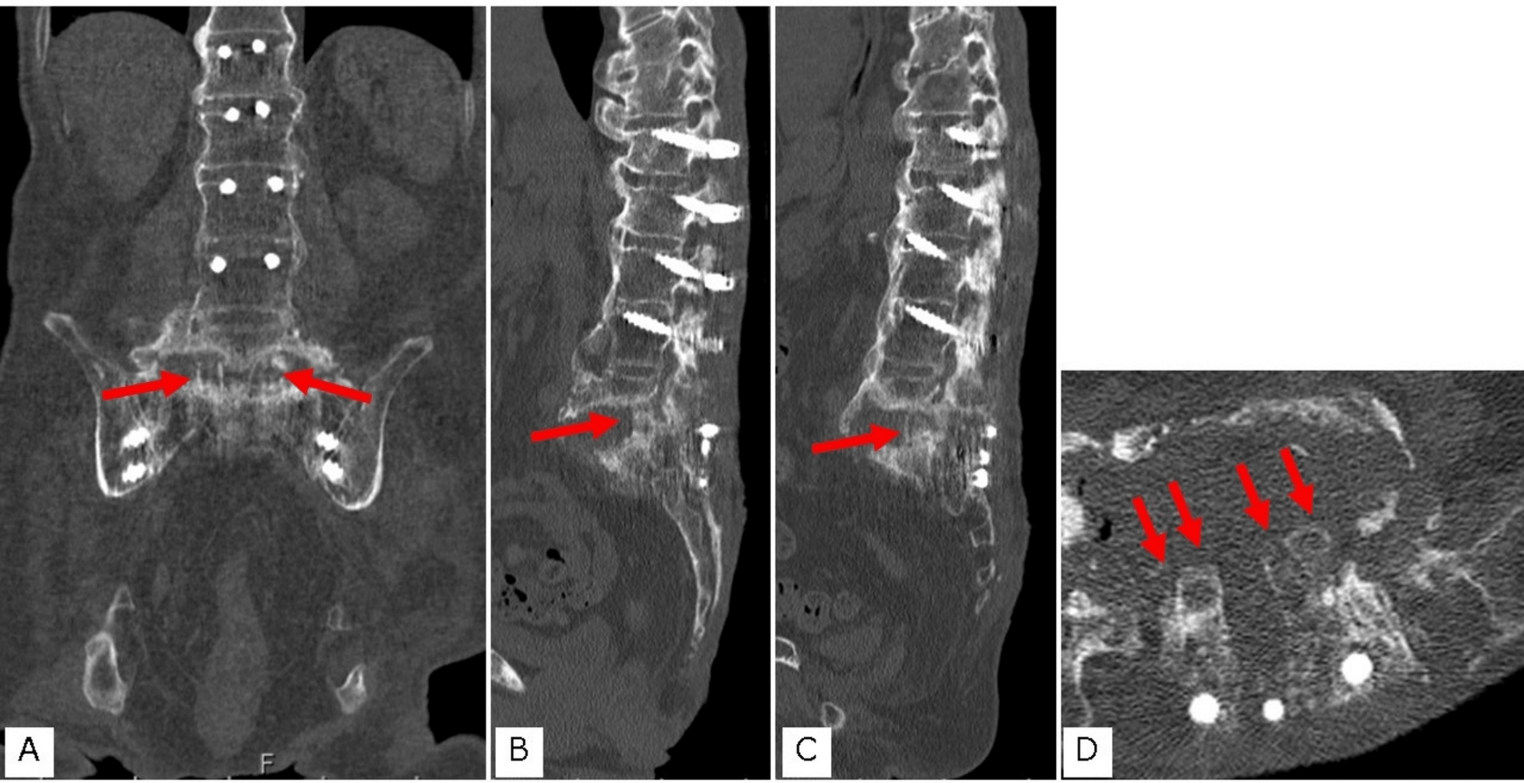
Computed tomography (CT) obtained six months after surgery A. Coronal plane; B and C. Sagittal plane; D. Axial plane at the L5/S1 level. The bone grafted in the intervertebral space and around the rods was not absorbed.
